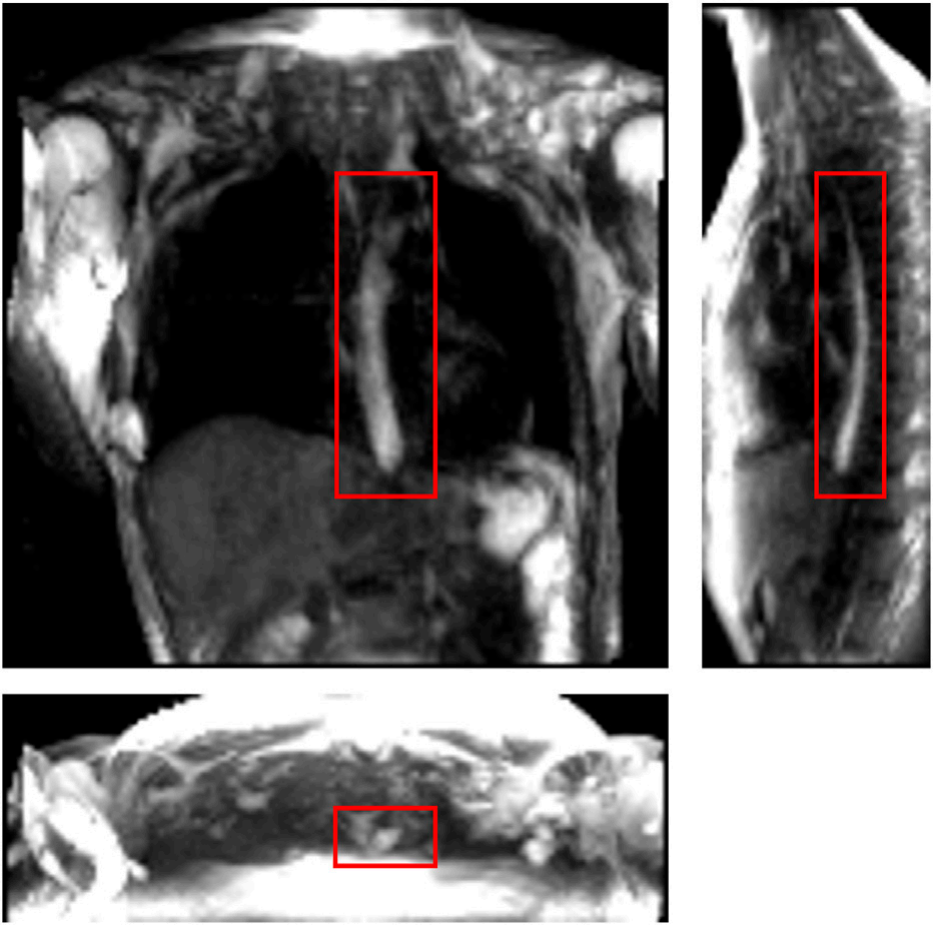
One instance of a dynamic MRI of a normal subject as seen in three perpendicular planes. The planes (from left to right to bottom) are coronal, sagittal, and axial, respectively. The bolus can be seen as the bright region inside the red boxes. Concentrated pineapple juice was swallowed as a contrast agent.
